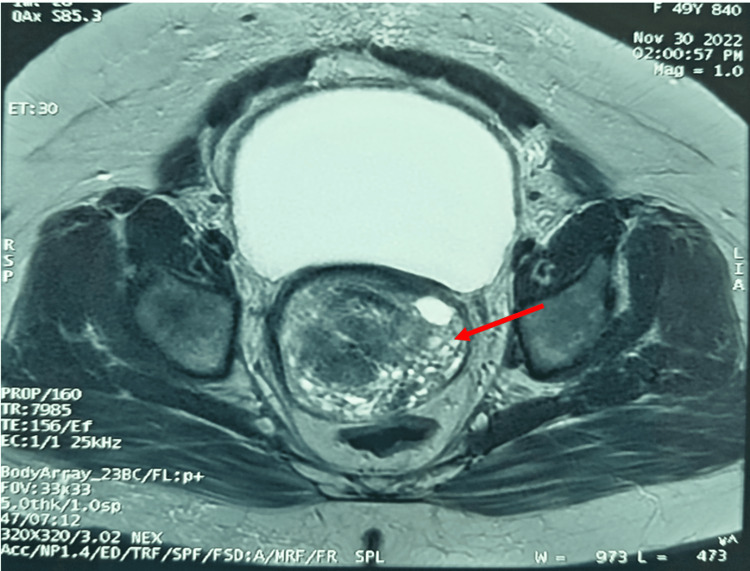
Axial T2-weighted pelvic MRI showing a large tumor mass occupying the uterine lumen (red arrow), consistent with a genital tract neoplasm
